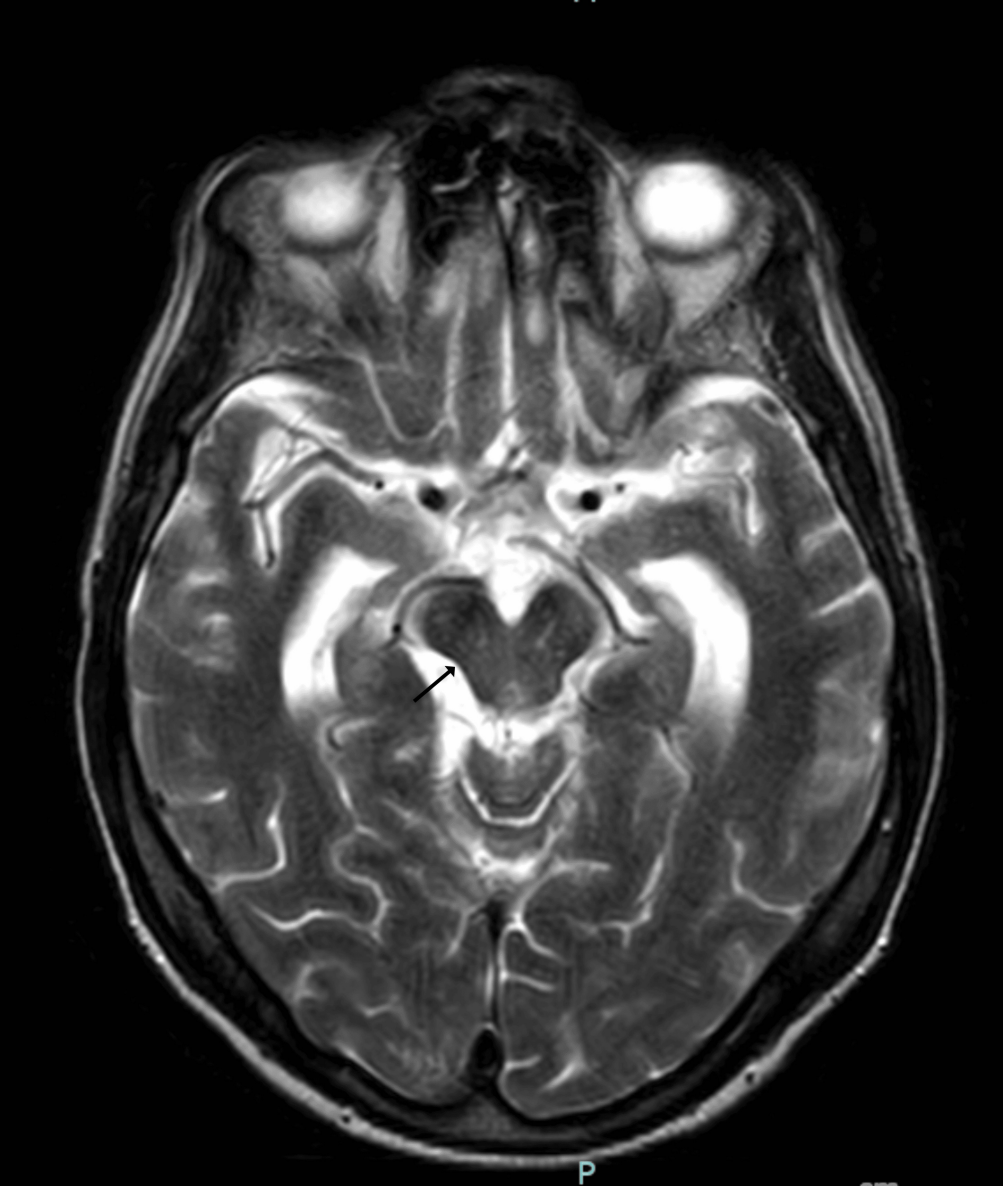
Midbrain atrophy. T2-weighted Turbo Spin Echo (TSE) axial MRI brain image showing midbrain atrophy (arrow).
